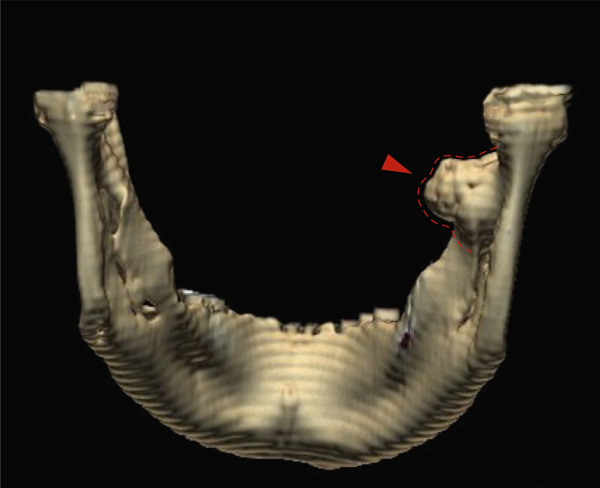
3D CT reformation of the mandible, showing the osteoma in the right ramus (arrowhead and dotted line).
